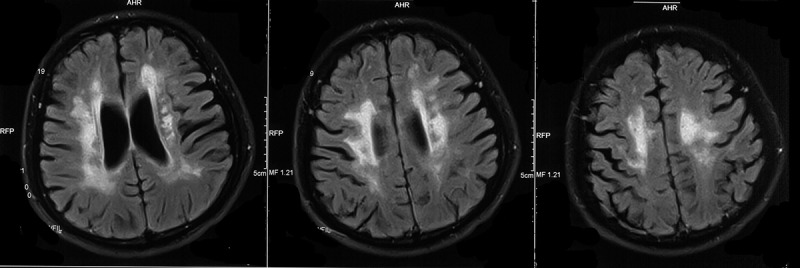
Head FLAIR and MRI images of the pro-band. Image shows bilateral lateral ventricles, bilateral semi-oval center with multiple abnormal signals, and demyelination in the white matter around the bilateral lateral ventricles.
